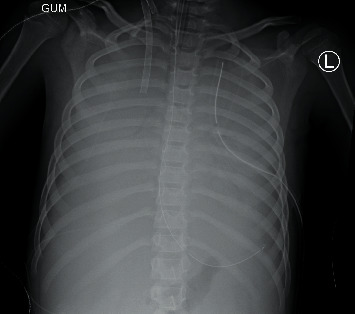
Chest radiograph showing progressive and complete opacification of bilateral lung fields.
